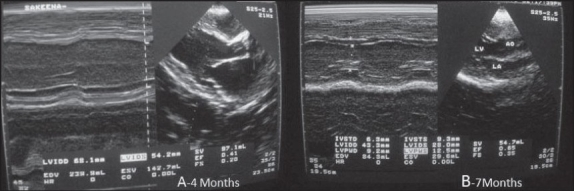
Echocardiographic left parasternal long axis view (2D and M mode) showing left ventricular dimensions and ejection fraction at four (A) and seven months (B) of treatment.
